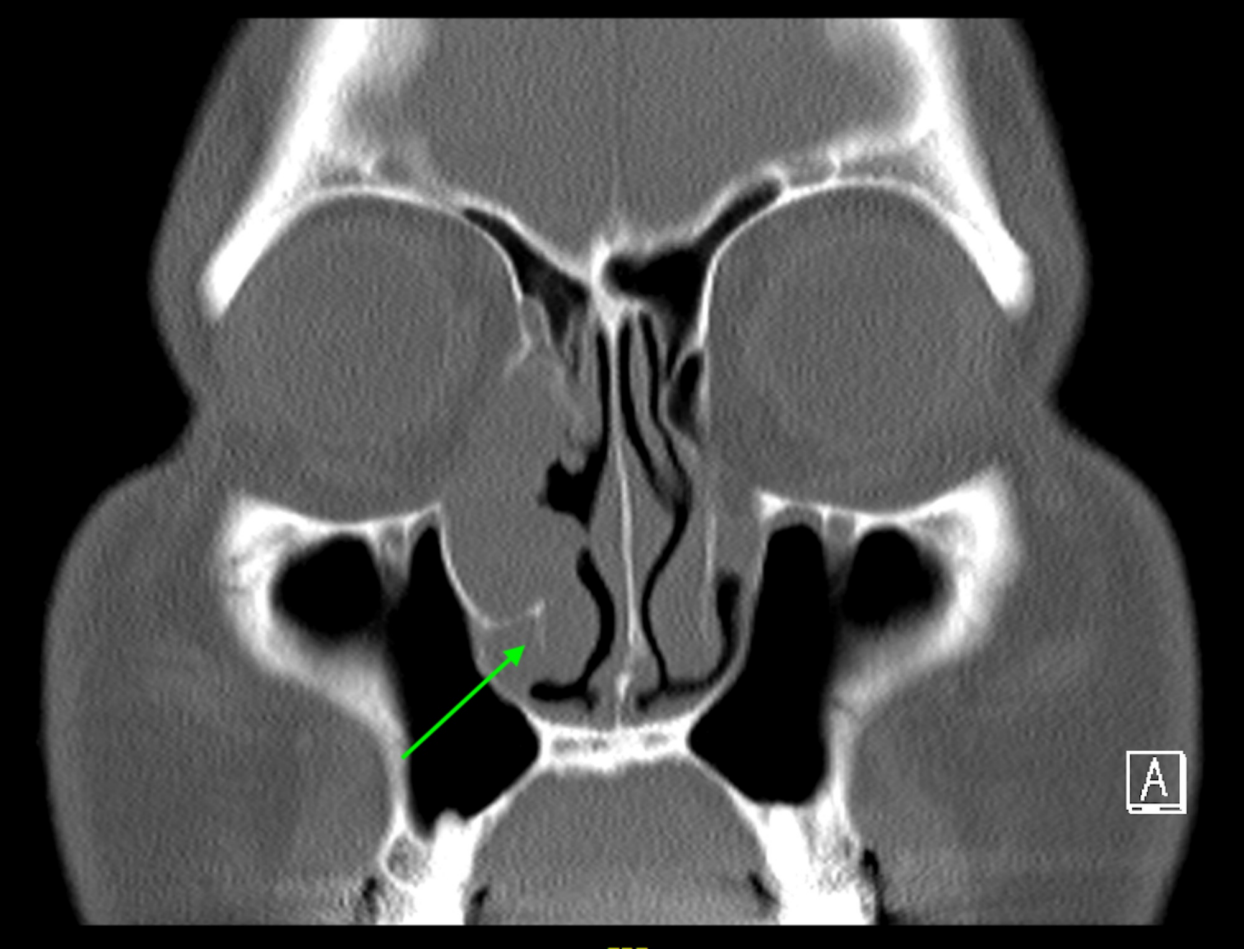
CT sinus done prior to first definitive surgical excision showing extension of mass into the inferior meatus.
